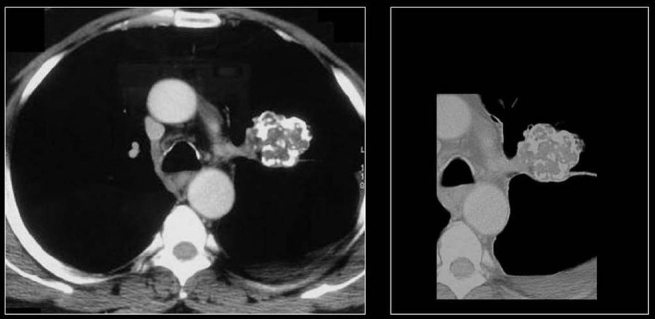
An axial CT scan just above the hila shows a large central PN with popcorn calcification in a hamartoma
